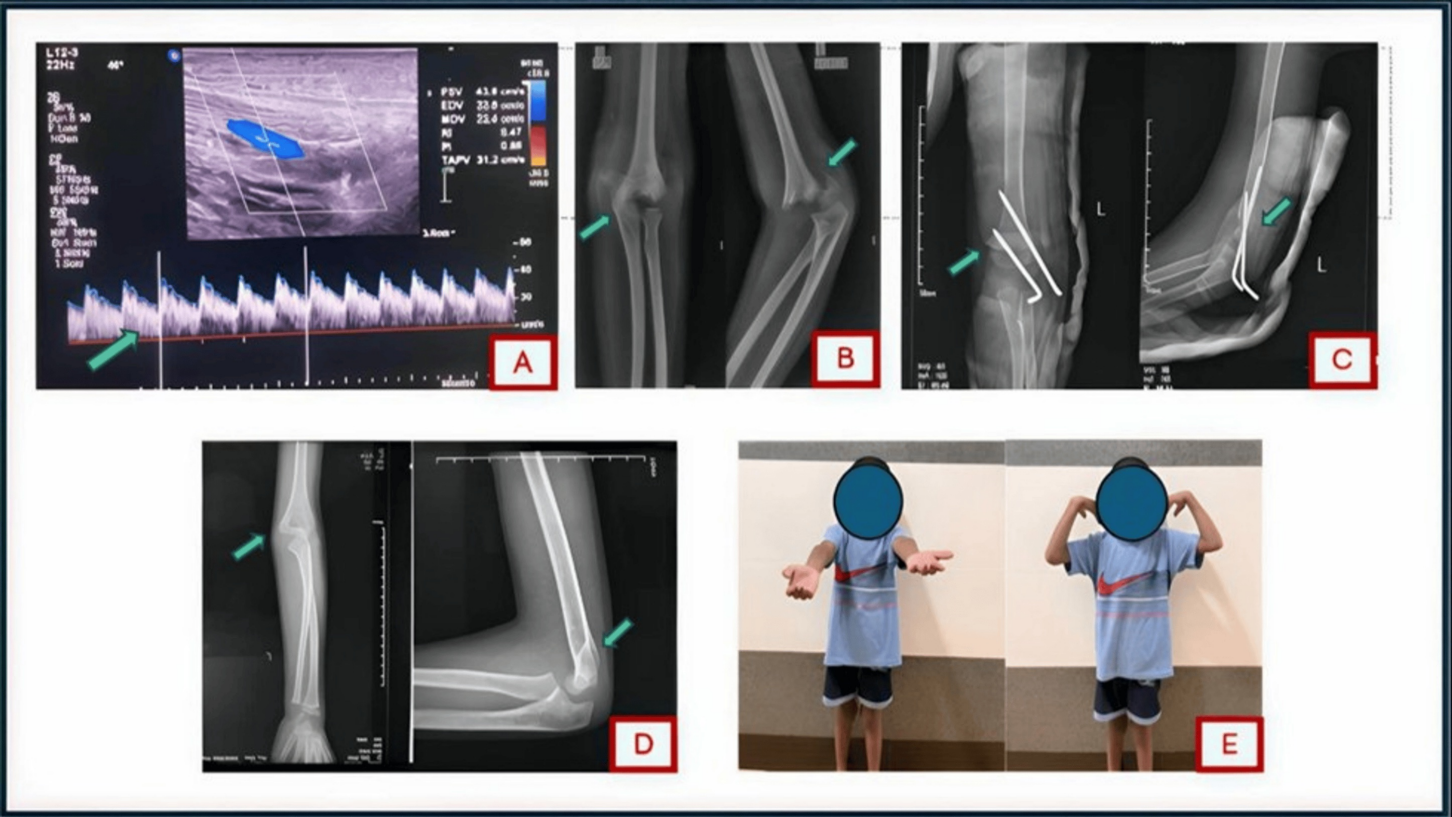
A nine-year-old boy with a Gartland type III displaced supracondylar humerus fracture presenting with a pink pulseless hand. (A) Color flow duplex ultrasound of the brachial artery shows (arrow) loss of the normal triphasic waveform (converted to a monophasic flow), indicating a brachial artery injury with maintained collateral perfusion. (B) Pre-reduction anteroposterior (AP) and lateral radiographs of the elbow demonstrating the completely displaced fracture (arrows). (C) Immediate post-reduction radiographs after closed reduction and percutaneous pinning, showing restoration of alignment (arrows). (D) Radiographs at 12 weeks post-operatively confirming successful fracture union (arrows) in good alignment. (E) Clinical photograph at 12 weeks demonstrating the patient’s regained full elbow range of motion.
